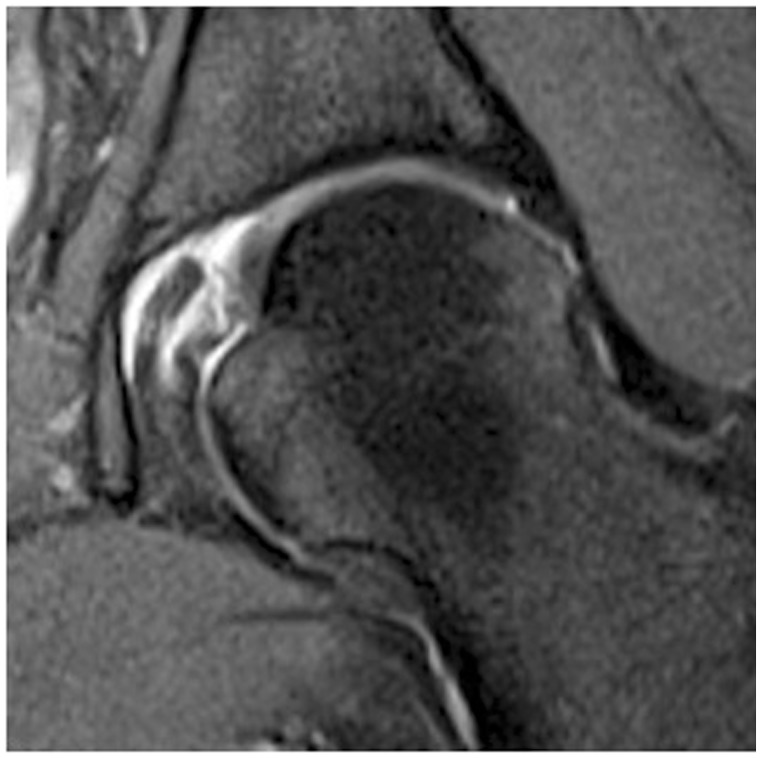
LT partial thickness tear-coronal oblique Proton Density MRI showing linear signal and intrinsic signal changes to foveal insertion of LT.
